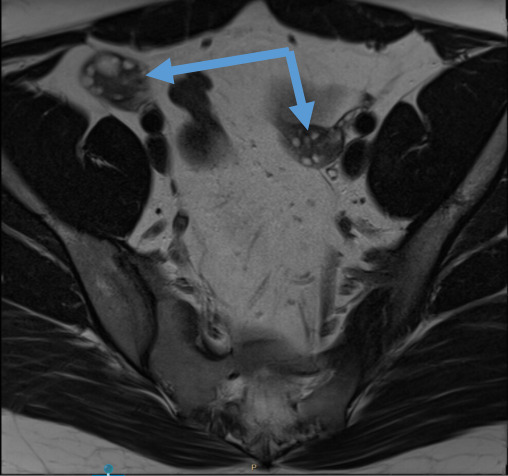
Pelvic MRI revealing normal ovaries (arrow).
